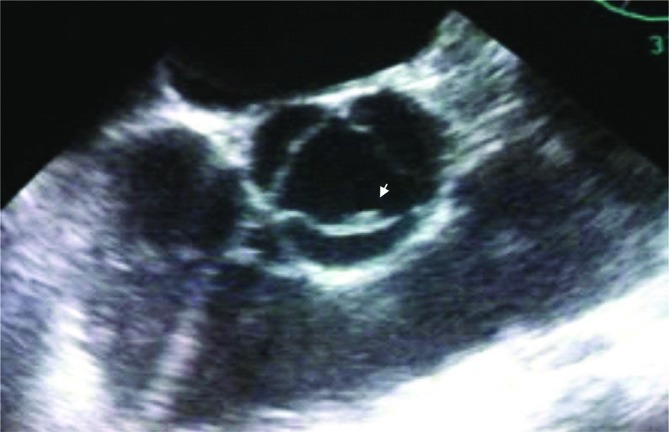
Infective endocarditis (IE) due to *Rhodotorula mucilaginosa.* A transesophageal echocardiogram showed a 0.3 cm thickening in the ventricular side of aortic valve (arrow).
